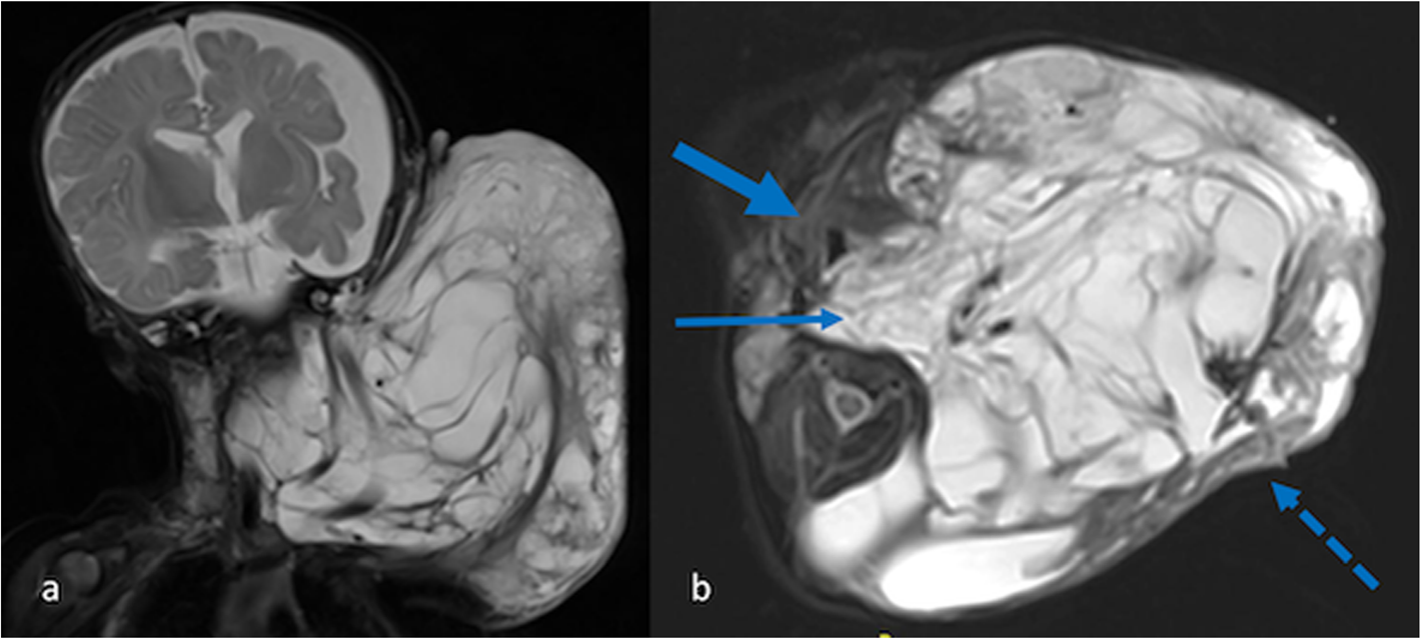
Lymphatic malformation in a 2 day old male. a coronal and b axial T2 weighted MR images delineate the complex lesion causing marked distortion of the anatomy of the neck. On the axial image, the prevertebral disease (thin arrow) displaces the trachea (thick arrow); this is the area that should be targeted with sclerotherapy to improve the child’s respiratory status. The more bulky, superficial disease (broken arrow), although tempting to treat, is of little clinical consequence at this stage
